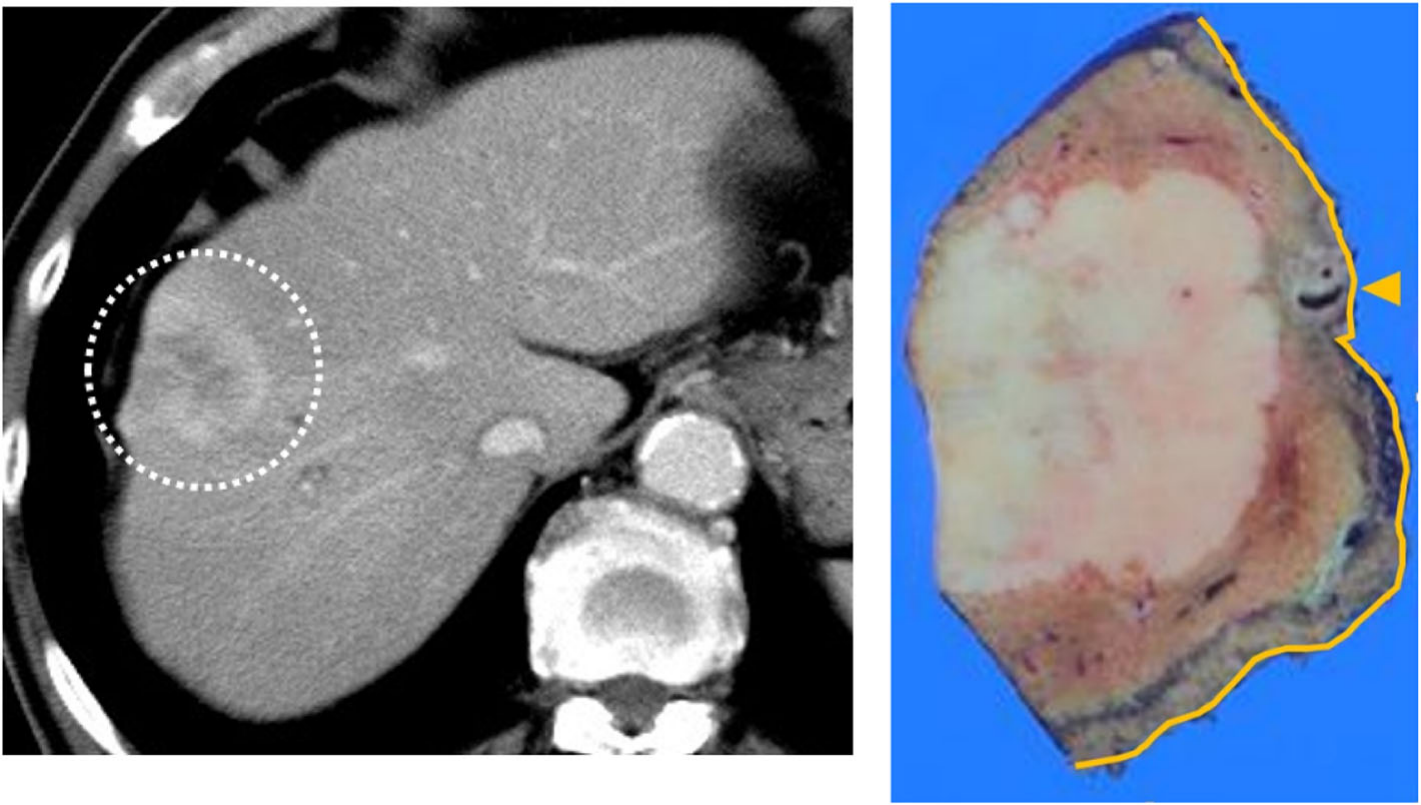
Computed tomography (axial image, white-dotted line circle) revealed an enhanced tumor. A macroscopic examination of the primary tumor revealed mass-forming type growth. The S8 Glisson stump (yellow arrow) and hepatic radial margin (yellow line) were tumor-free
